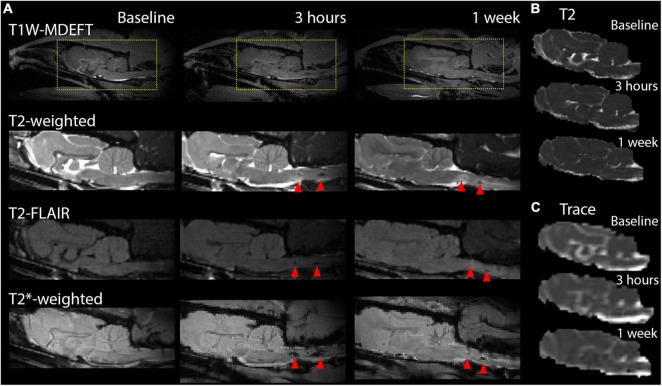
Markers of pathology on contrast weighted MRI scans, T2, and diffusion maps. Conventional MRI images for T1, T2, and T2* contrasts are shown at the sagittal midline prior to TBI and at 3 h and 1 week after to demonstrate the MRI profile of focal damage (red arrows) in this model including T2* hyperintensities post-TBI and T2-weighted hyperintensities at 1 week following severe injury. T2 (B) and DTI trace (C) maps are shown for the same brain and time points but do not feature strong focal abnormalities in the region of map coverage.
